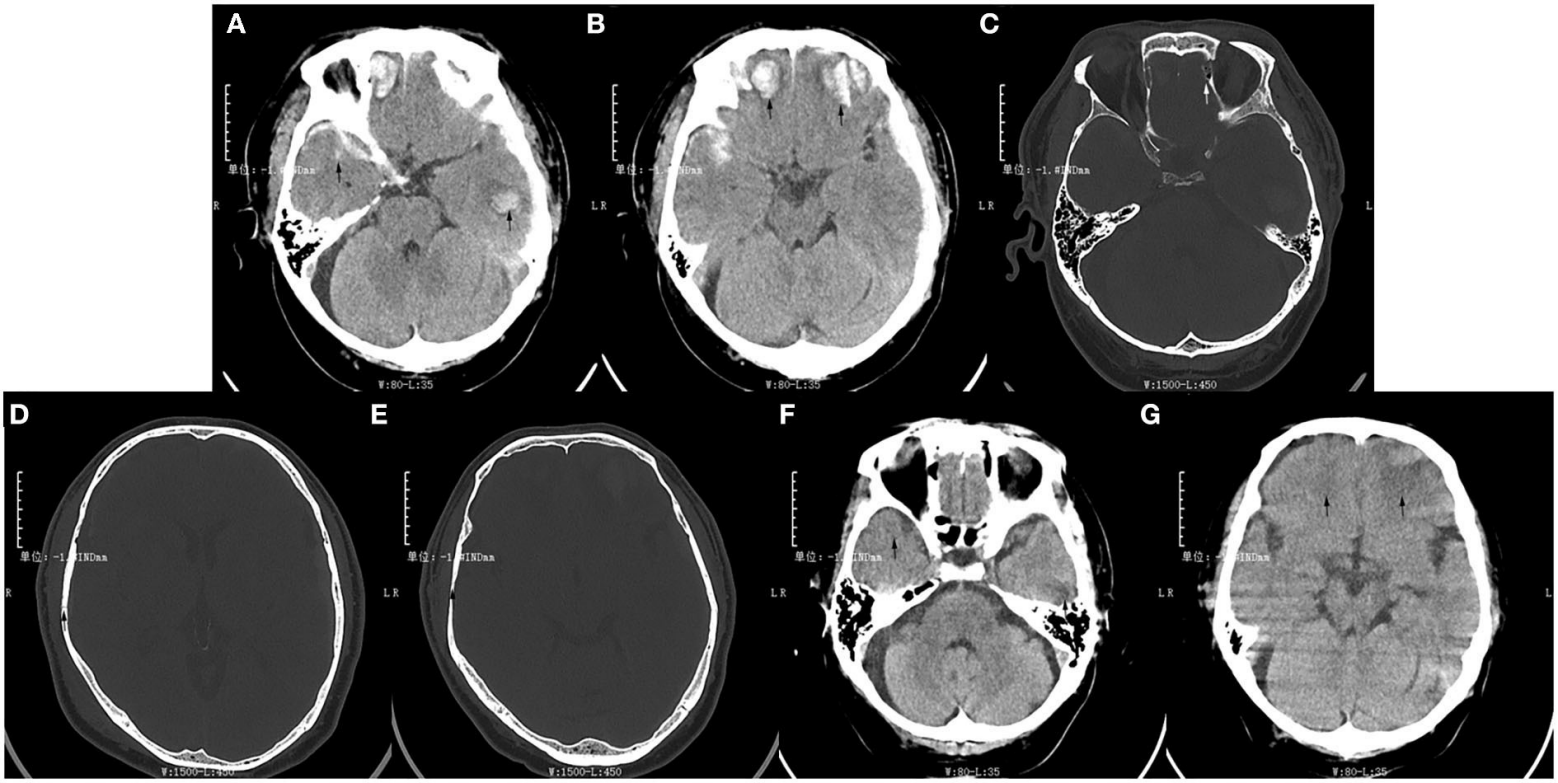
The patient's brain computed tomography results of the first hospitalization after trauma. (A,B) Two pieces of high-density signal were, respectively, in the bilateral temporal lobe and frontal lobe at the time of admission (arrows). (C) The continuity of the left orbital bone was interrupted (arrow). (D,E) The continuity of the right cranial parietal bone and temporal bone were interrupted (arrows). (F,G) The signal of lesion area displayed low-density in the bilateral temporal lobe and frontal lobe at the time of discharge (arrows).
